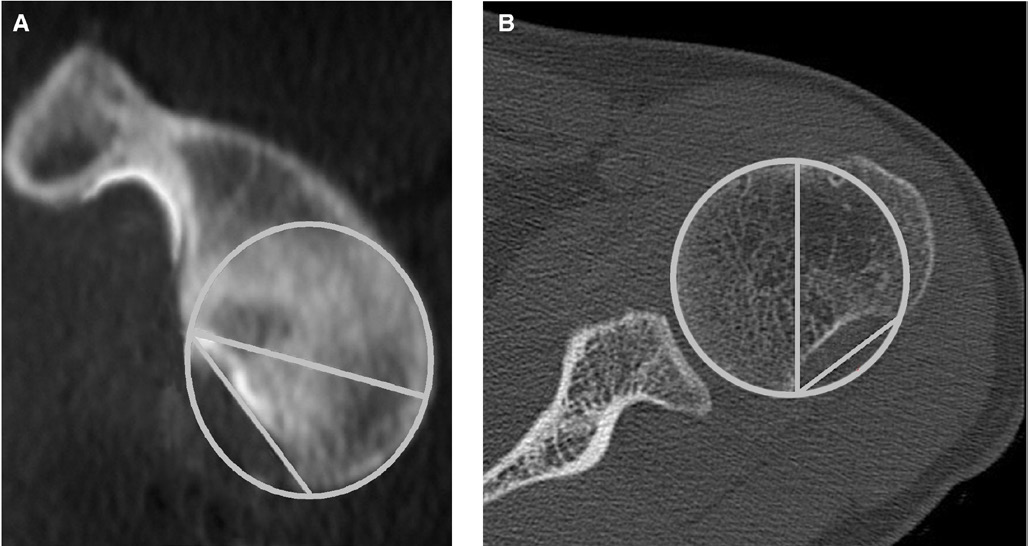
(A) The glenoid bone defect is considered significant when the tangential length of the defect is equal or more than 50% of the maximal width of the glenoid surface on a two-dimensional en face CT view.^29^ (B) The humeral defect is considered significant when the maximal width of the Hill-Sachs defect is equal or more than 40% of the diameter of the humeral head on a two-dimensional axial CT view.^30 31^
